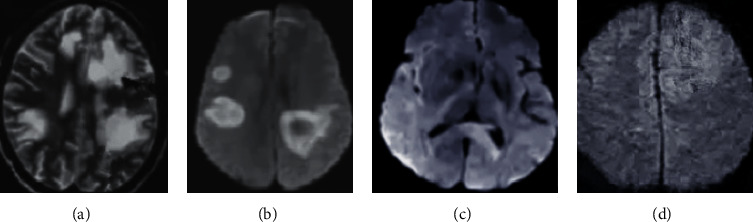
Partial MRI results of stroke patients. (a) TI; (b) T2; (c) T1C; and (d) flair.
